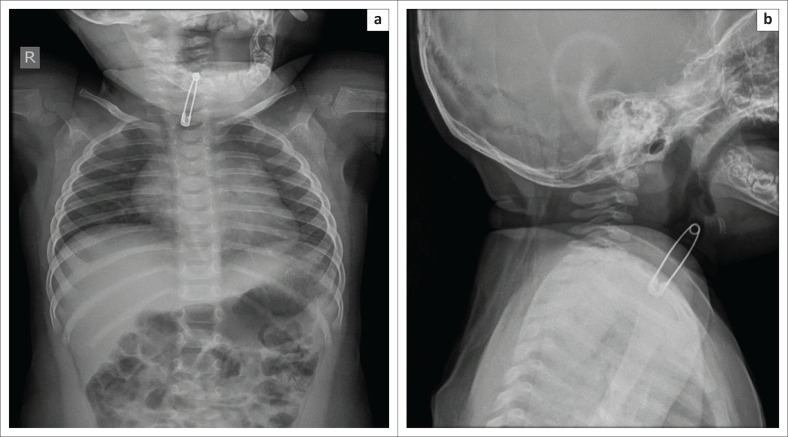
Frontal (a) and lateral (b) radiographs of the neck in a 2-year-old child with an aspirated safety pin in the subglottic region.
